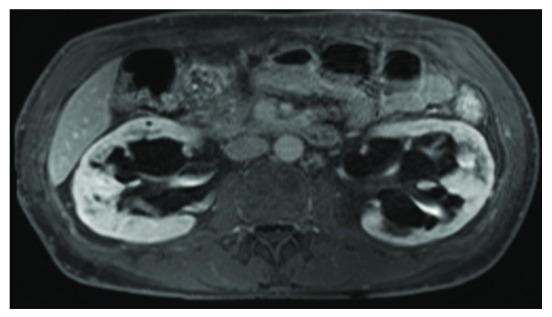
Parapelvic cysts in Fabry disease. MRI scan of proband from family C demonstrating bilateral parapelvic cysts.
